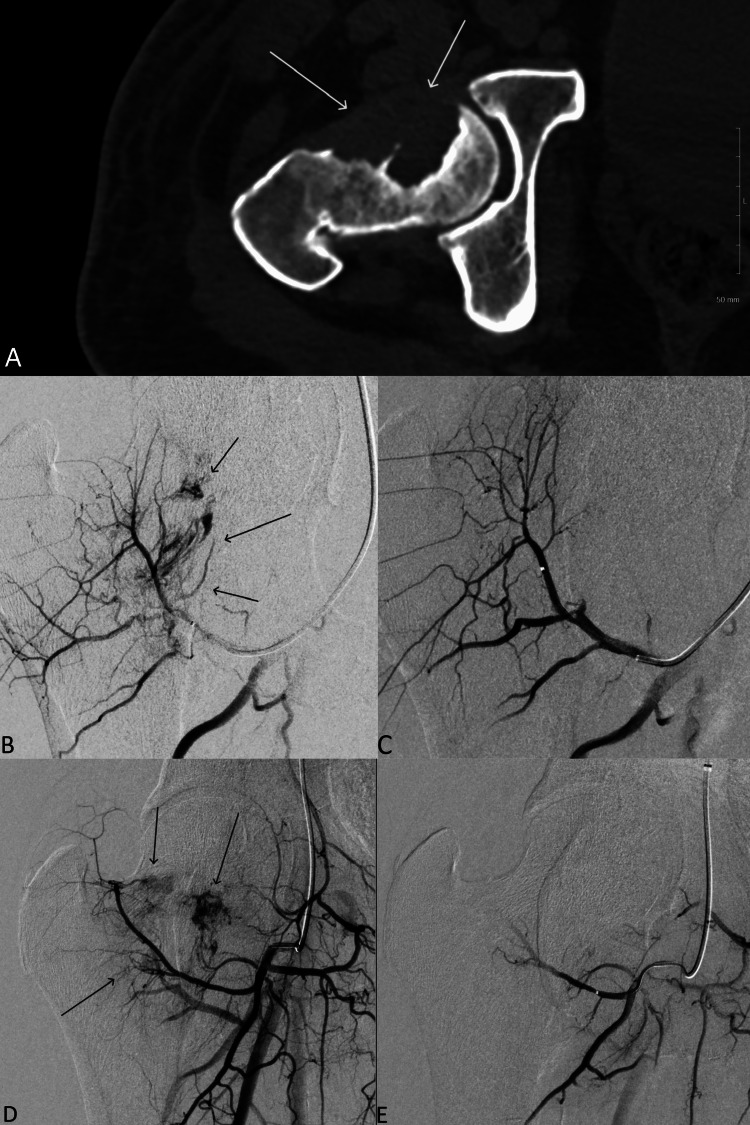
A 52-year-old male with bilateral lower extremity pain and weakness. A) Axial CT of the hip demonstrating lytic destruction of the right femur (white arrow). B) D) Digital subtraction angiography (DSA) demonstrating areas of tumor blush supplied by the branches of the perforating femoral and the left lateral circumflex arteries (black arrows). C) E) DSA demonstrating results of embolization.
